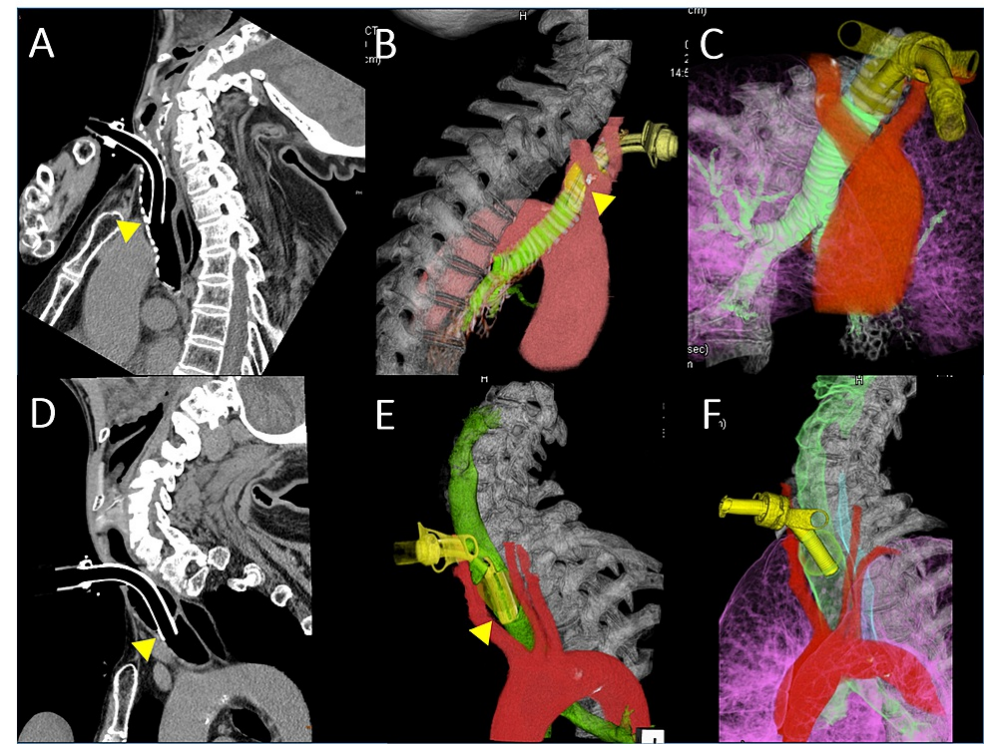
Neck-thoracic CT Computed tomography findings. (A-C) Case 1 with Parkinson’s disease. (A) The neck is in a severe extended position (retrocollis). (B) Colored and 3D-reconstructed images show that the tip of an ordinary silicone tracheostomy tube has attached to the tracheal anterior wall, where the innominate artery transverses (arrowhead). (C) After switching to an adjustable tracheostomy tube, CT shows that the tip of the adjustable tracheostomy tube is in a safe position away from the tracheal anterior wall. (D-F) Case 2 with multiple system atrophy. (D) Case 2 also shows severe retrocollis. (E) The tip of an ordinary silicone tracheostomy tube has attached to the tracheal anterior wall across the innominate artery (arrowhead). (F) After switching to an adjustable tracheostomy tube, the tip of the adjustable tracheostomy tube is confirmed to be in a safe position.
